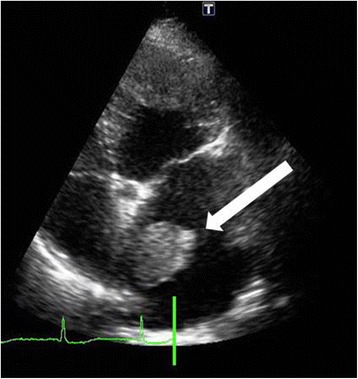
Transthoracic echocardiography showing a 33 × 30-mm mass in the left atrium (white arrow)
